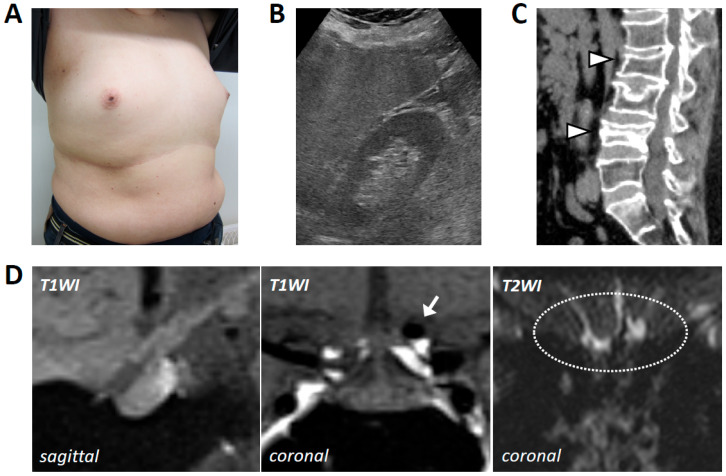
Clinical presentations of the patient with a novel variant of *FGFR1*. (A) The patient showed gynecomastia and loss of axillary and pubic hair. (B) Abdominal ultrasonography revealed findings of fatty liver. (C) Spinal CT showed compression fractures of the second and fourth lumbar vertebrae (shown by arrowheads). (D) Sagittal (left), coronal T1-weighted (center), and coronal T2-weighted MRI images of the patient. The MRI revealed no abnormalities in the hypothalamus or pituitary gland, but an internal carotid artery aneurysm was incidentally detected (shown by an arrow), and loss of the olfactory bulb was observed (shown by a circle).
